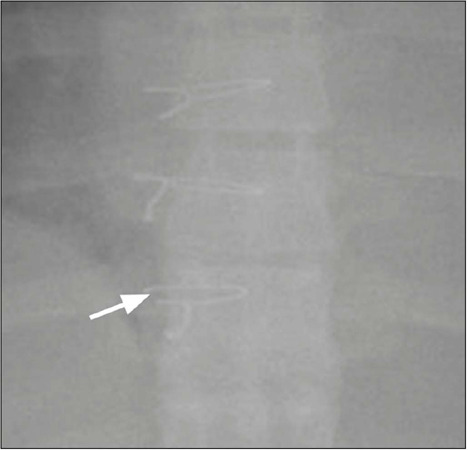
Sternal wire fracture (magnified chest X-ray image): discontinuity on
